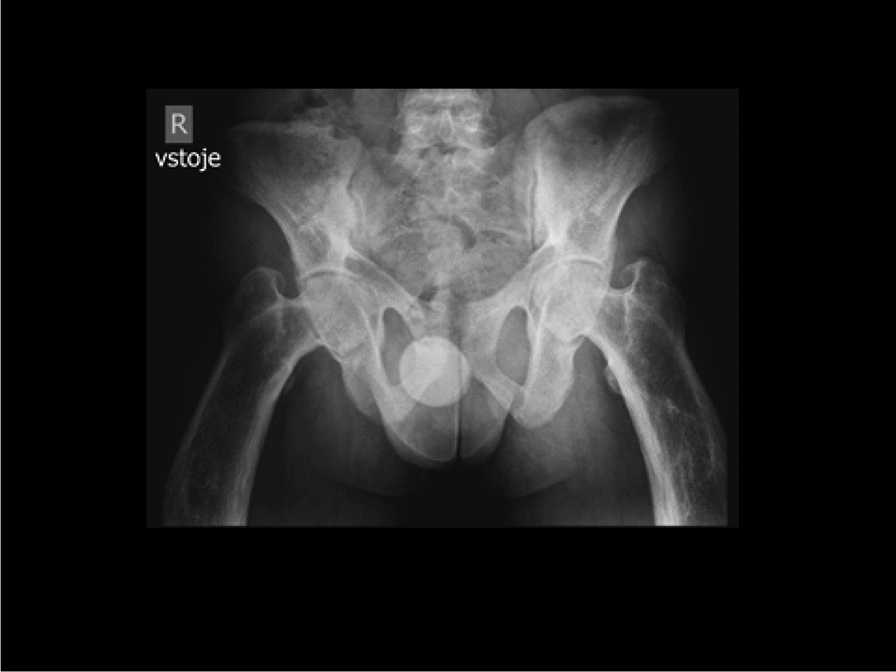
AP radiography of the pelvis (age 20 Y). Diffuse osteosclerosis of the pelvis and both femurs, bilateral acetabular protrusion and varus deformity of both femoral necks can be seen. The proximal diaphyses of femurs are widened due to widespread periosteal appositions, with coarse trabeculae of the spongy bone and with varus deviation
